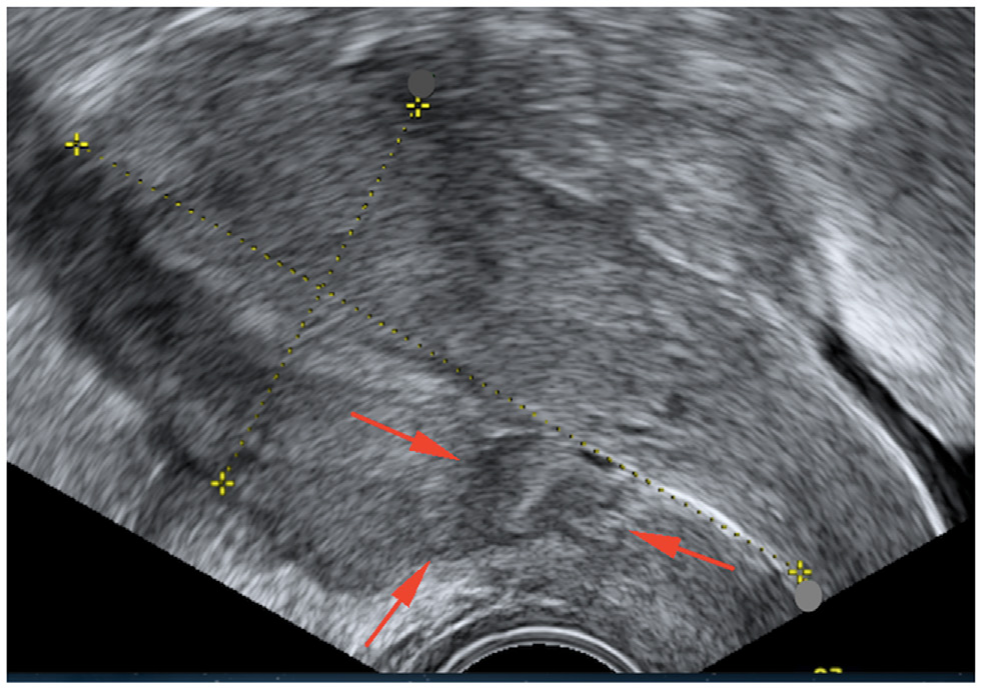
Pelvic ultrasonography after medical treatment shows a small residual heterogenic cavity at the site of cesarean section.
